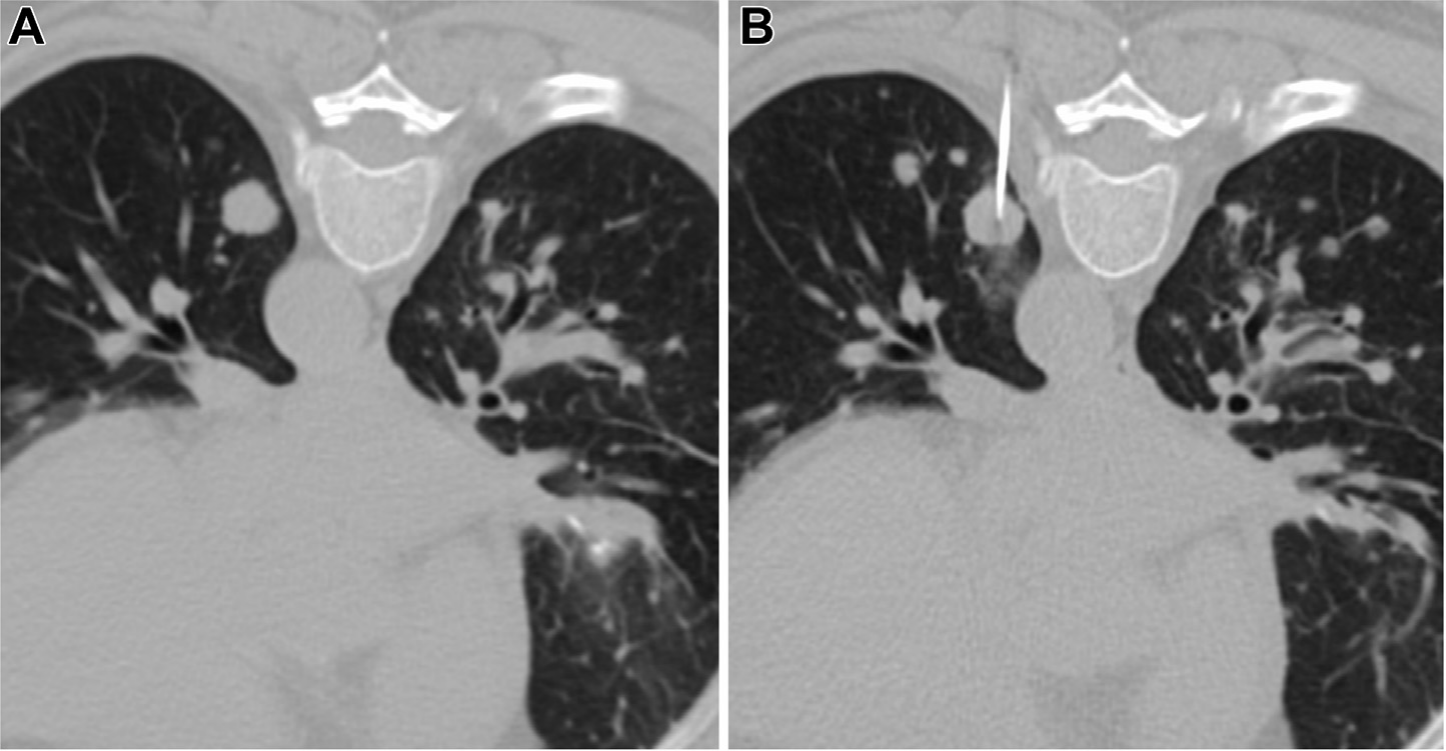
A 59-year-old male with a history of metastatic lung adenocarcinoma. *(A)* Preprocedural diagnostic CT shows innumerable diffuse bilateral pulmonary nodules. *(B)* The largest nodule (<3 cm) in the left lower lobe was targeted at its center in which seven core biopsies were obtained followed by injection of CCL21 dendritic cells. CT, computed tomography.
